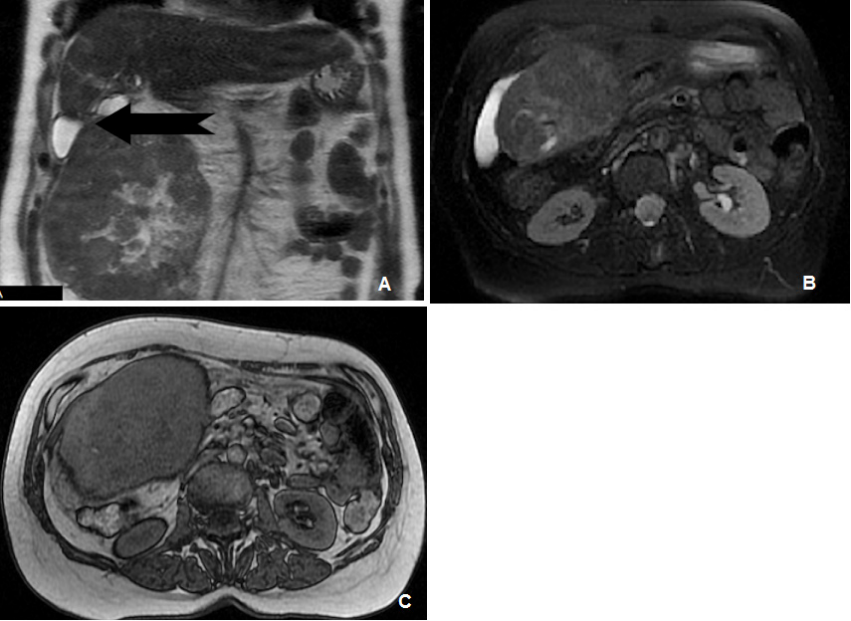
MRI images of the pedunculated tumor. A: MR T2 coronal image showing a heterogeneous tumor mass underneath the right hepatic lobe. Arrow indicates tumor pedicle. B: MR T2 fat suppressed axial image depicting a heterogeneous signal intensity liver mass. C: MR T1 axial image showing a large right liver lobe mass displacing intestinal loops.
